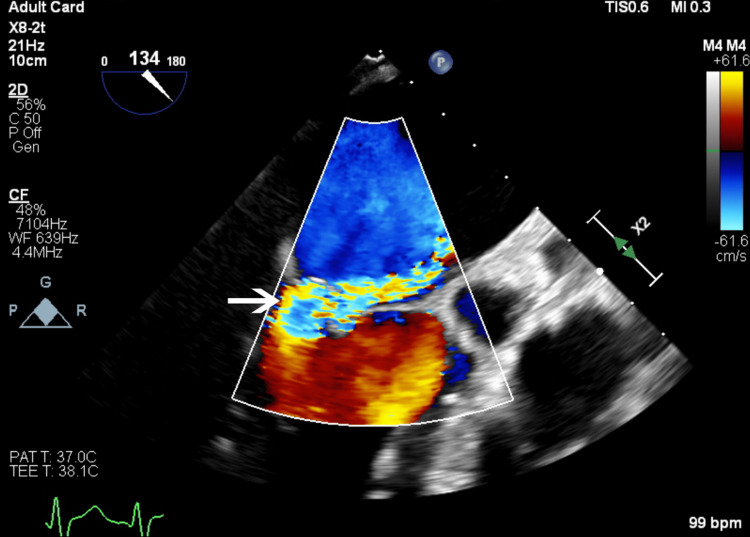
Transesophageal echocardiography image demonstrating a two-dimensional view of mitral regurgitation jet in color Doppler.
